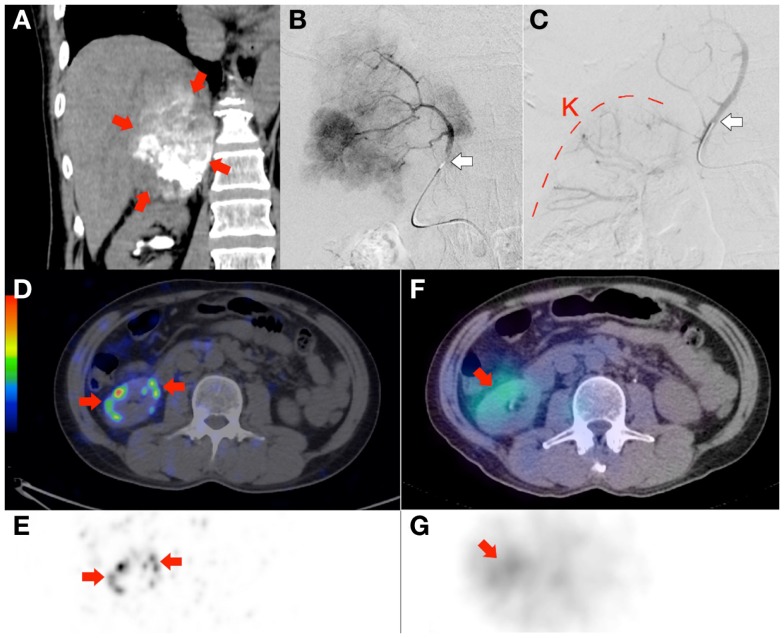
Radioembolization to a site other than the liver. Bulky right adrenal metastasis from chemorefractory gastrointestinal stromal tumor. (A) Catheter-directed CT angiography of the right inferior adrenal artery delineates the tumor (arrows) and the target arterial territory. (B) Digital subtraction angiogram (DSA) immediately prior to microsphere injection with the catheter tip (arrow) deep within the right inferior adrenal artery demonstrates good forward flow of contrast toward the tumor. (C) Post-RE DSA with no change to catheter tip (arrow) position demonstrates significant vascular stasis and reflux of contrast down the right inferior adrenal artery and distally into the terminal branches of the right renal artery. The renal cortex (curved dashed line) of the right kidney (“K”) can be seen. (D,E)
